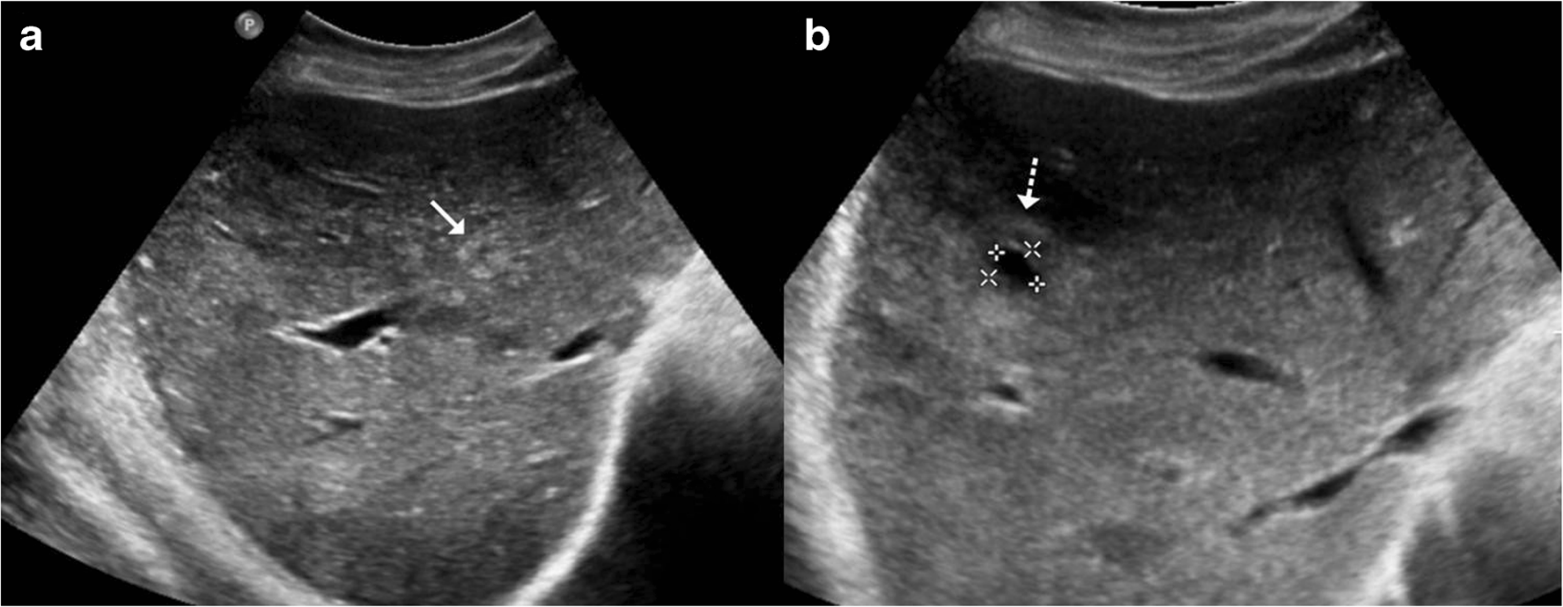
a Hepatic tuberculosis manifesting as hyperechoic liver lesions on ultrasonography (*arrow*). b One of the larger lesions shows central caseation and necrosis (*dotted arrow*)
